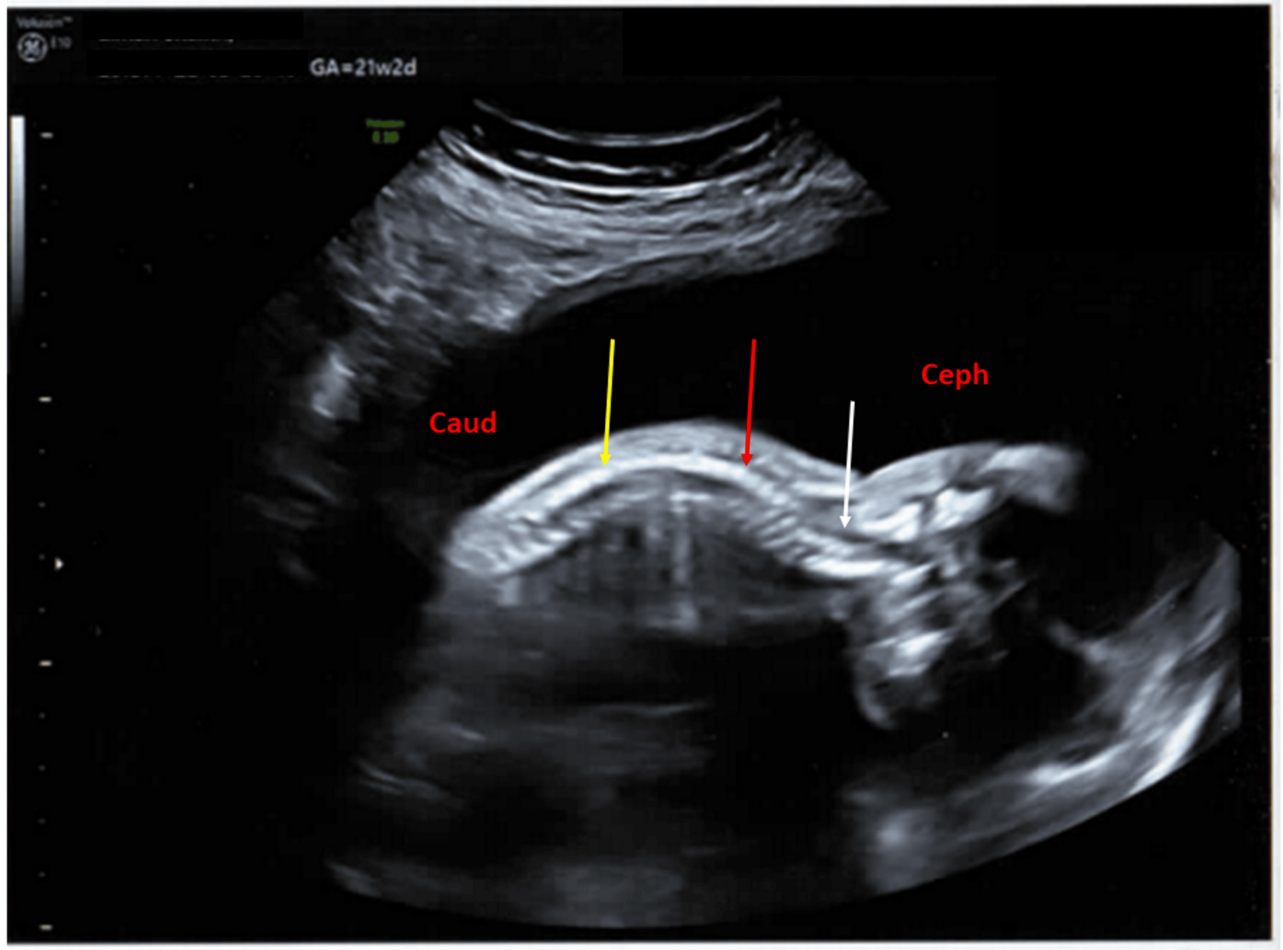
Antenatal ultrasound of the fetus in the second pregnancy showing the fetal spine Antenatal ultrasound of the fetus in the second pregnancy at around 21 weeks 2 days gestation, showing a sagittal view of the spine (ceph: cephalic side, caud: caudal side) with normal anatomy; the cervical (white arrow), thoracic (red arrow), and lumbar (yellow arrow) segments are seen with normal curvature.
